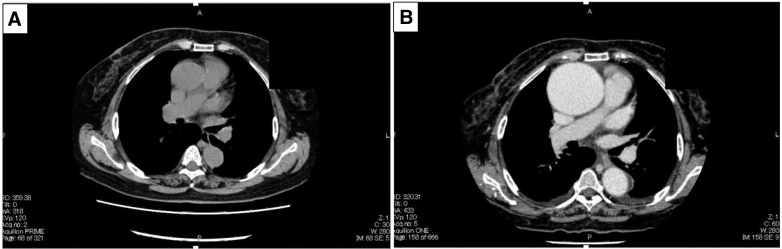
Three years ago (A) and just before surgery (B), preoperative computed tomography image showed that the diameter of the aorta was 41 mm and 71 mm.
